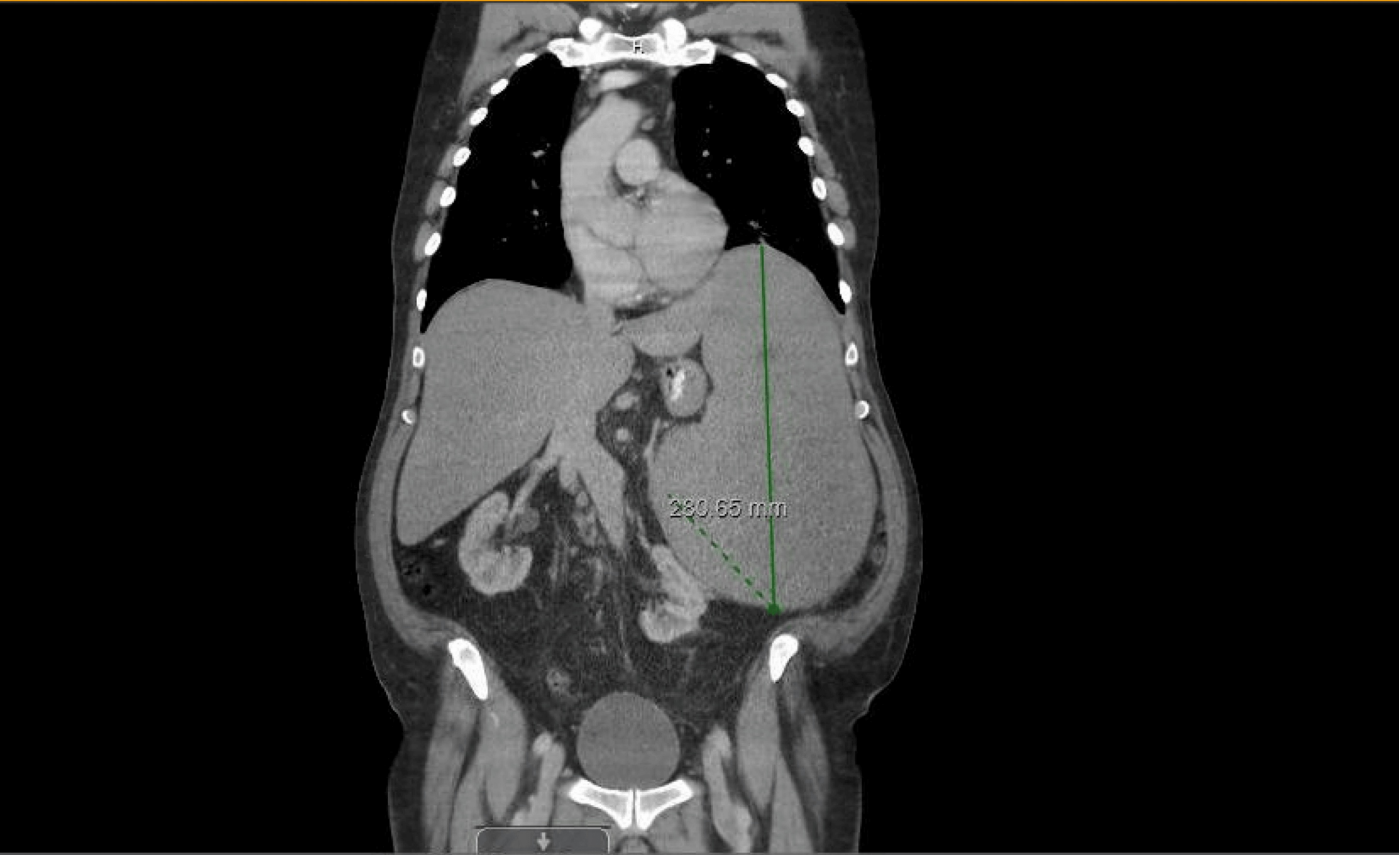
CT scan of the chest, abdomen, and pelvis showing hepatomegaly and splenomegaly, with the spleen measuring 28 cm.
